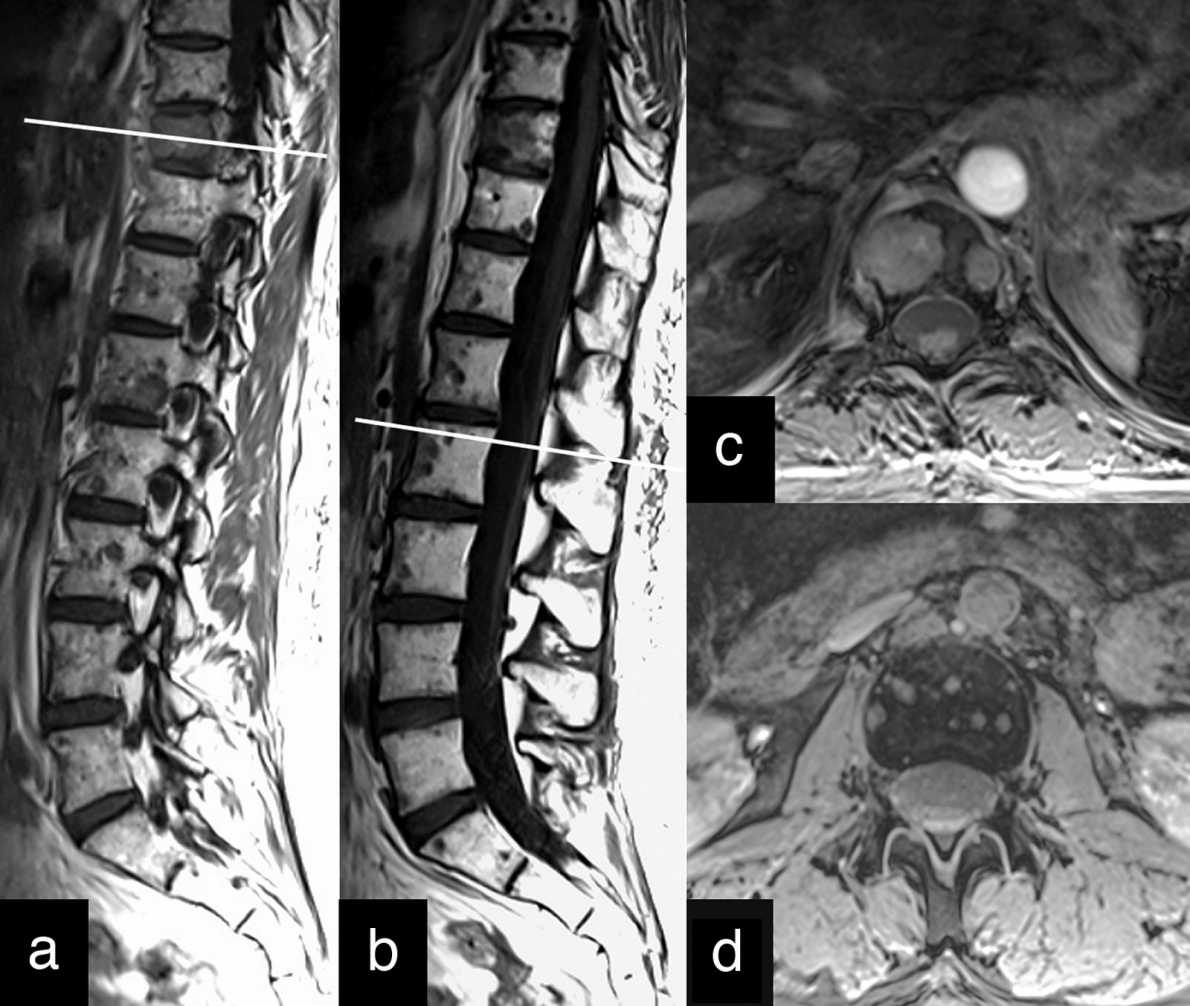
3-Tesla MRI of the spine discovers uncountable tiny metastatic lesions of the entire spinal column A: T1-weighted paramedian section. B: T1-weighted midsagittal section. C: T1-weighted axial section at the level indicated on a shows contrast enhancement of two gross metastases in Th10 vertebral body. D: T1-weighted axial section at the level indicated on B demonstrates multiple small metastases in L2 vertebral body
